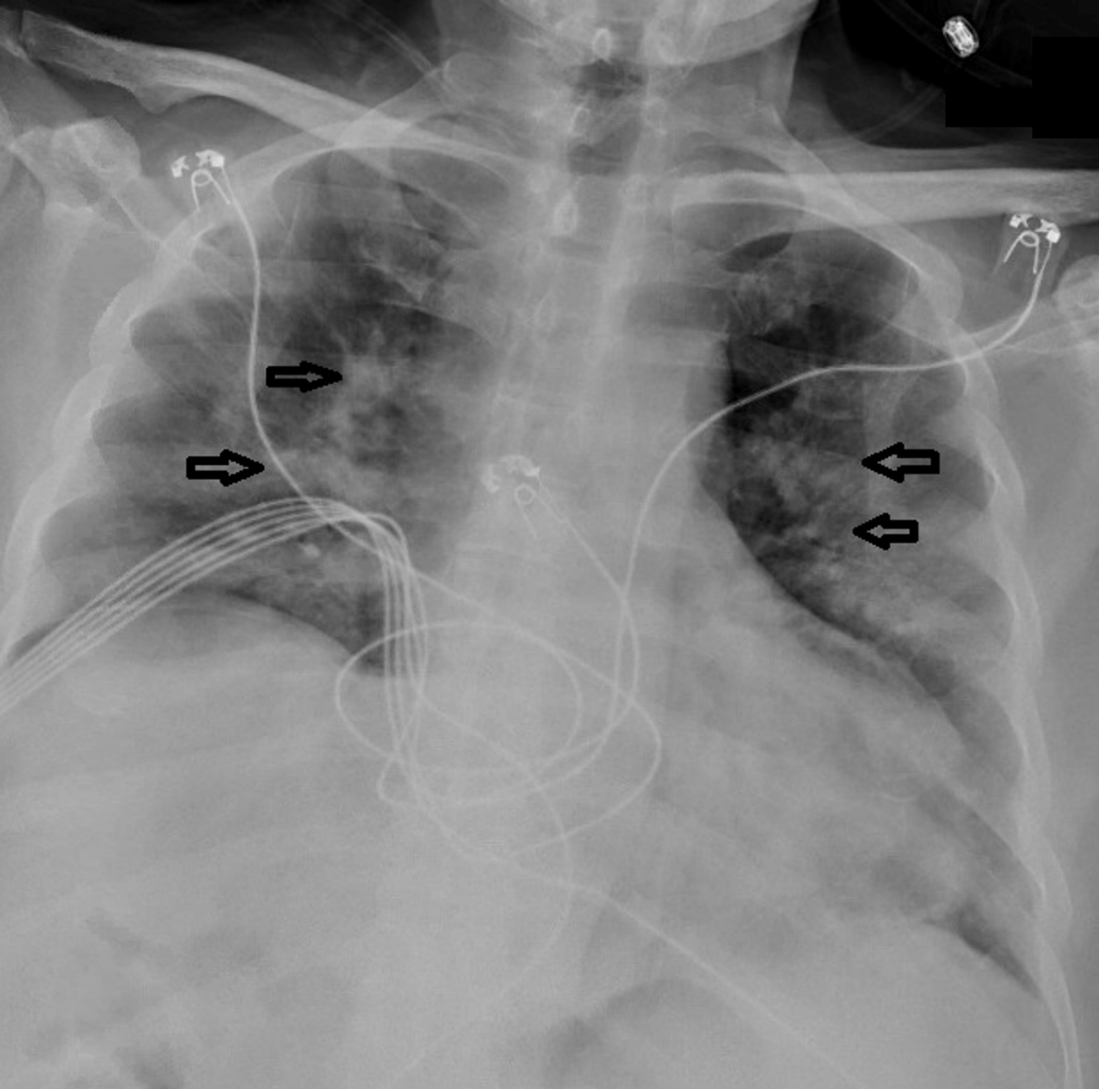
Portable x-ray (chest; AP view) showing bilateral pulmonary infiltrates compatible with COVID-19 pneumonitis. AP, anteroposterior
